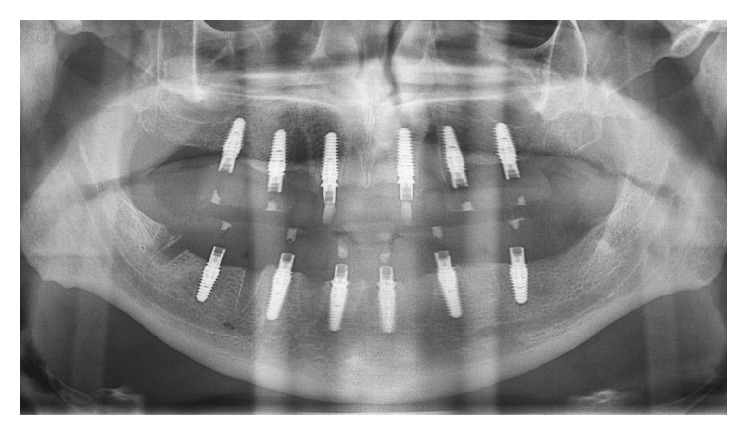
Panoramic radiograph taken 72 hours after surgery, when the implants were functionally loaded with the temporary acrylic resin FFA prostheses.
